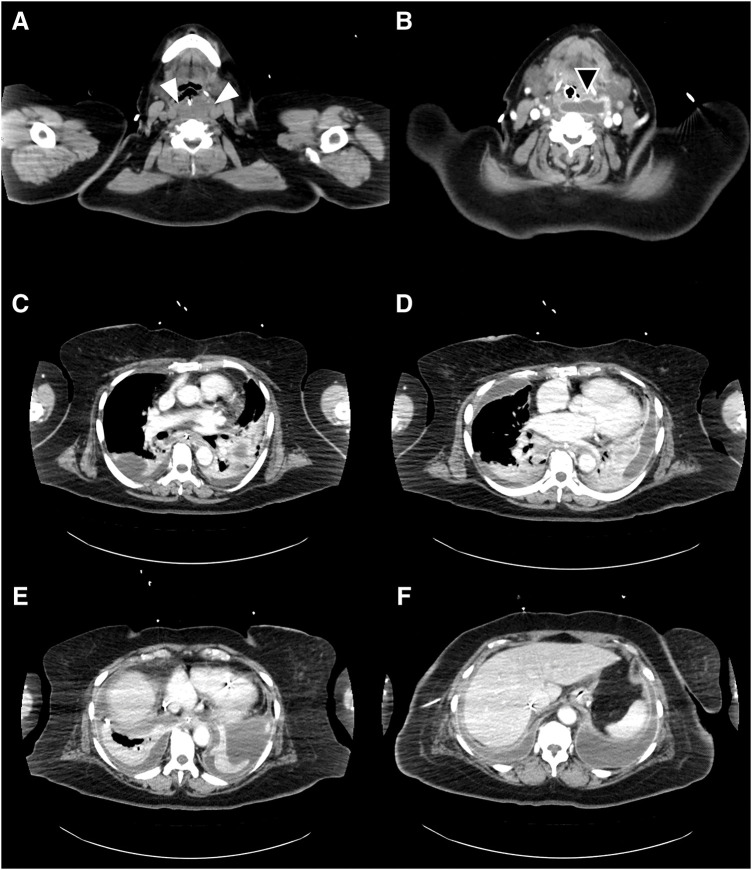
Enhanced CT scan serial transverse planes from the cervical region to the spleen before emergency surgery. (A) At the lower pharyngeal level, a low-density mass is noted (white triangles). (B) At the cervical esophageal level, an abscess with an enhanced capsule was noted (black triangle). (C) At the tracheal bifurcation level, pleural effusion is noted in both thoracic cavities. (D) Pleural effusion was noted on the left side of the thoracic cavity at the right atrial level. (E) At the EGJ level, massive pleural effusion causing retraction of the inferior lung lobes was noted in both thoracic cavities. (F) In the upper portion of the spleen, the left side of the thoracic cavity appeared continuous with a linear stapler. EGJ, esophagogastric junction
